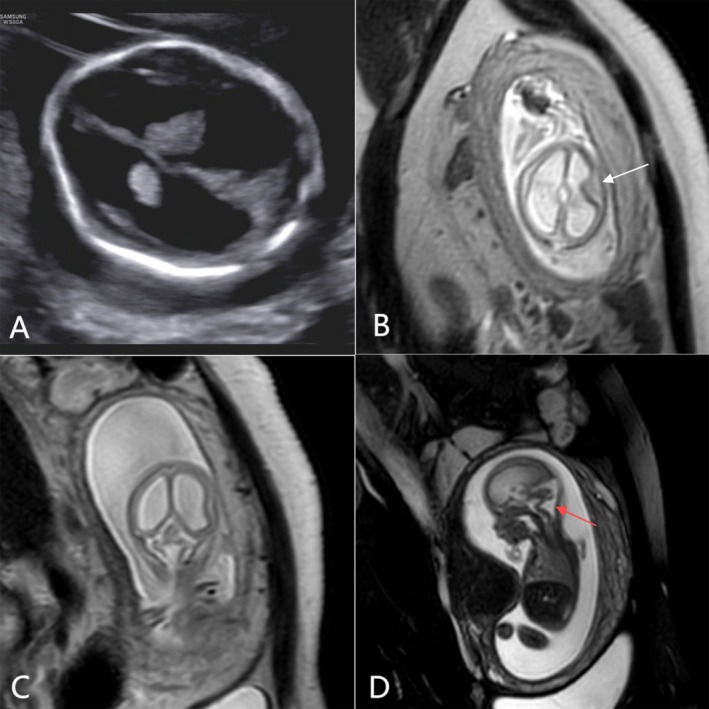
Images from Case 2. (A) US imaging at 17 weeks and 2 days reveals severe hydrocephalus in the lateral ventricle (arrow). (B) In vivo MRI at 17 weeks and 5 days, with axial T2—weighted imaging, demonstrates agyria and the absence of the pericerebral space, resulting in interhemispheric pseudofusion. Severe ventriculomegaly is again associated with periventricular cortical nodular heterotopias, a manifestation of a neuronal migration disorder (arrow). (C) A midline sagittal T2—weighted image shows severe hydrocephalus in the lateral ventricle. (D) The brainstem is hypoplastic and characterized by a dorsal pontomesencephalic kink (red arrow). The third ventricle is dilated, and the corpus callosum is hypoplastic yet intact.
